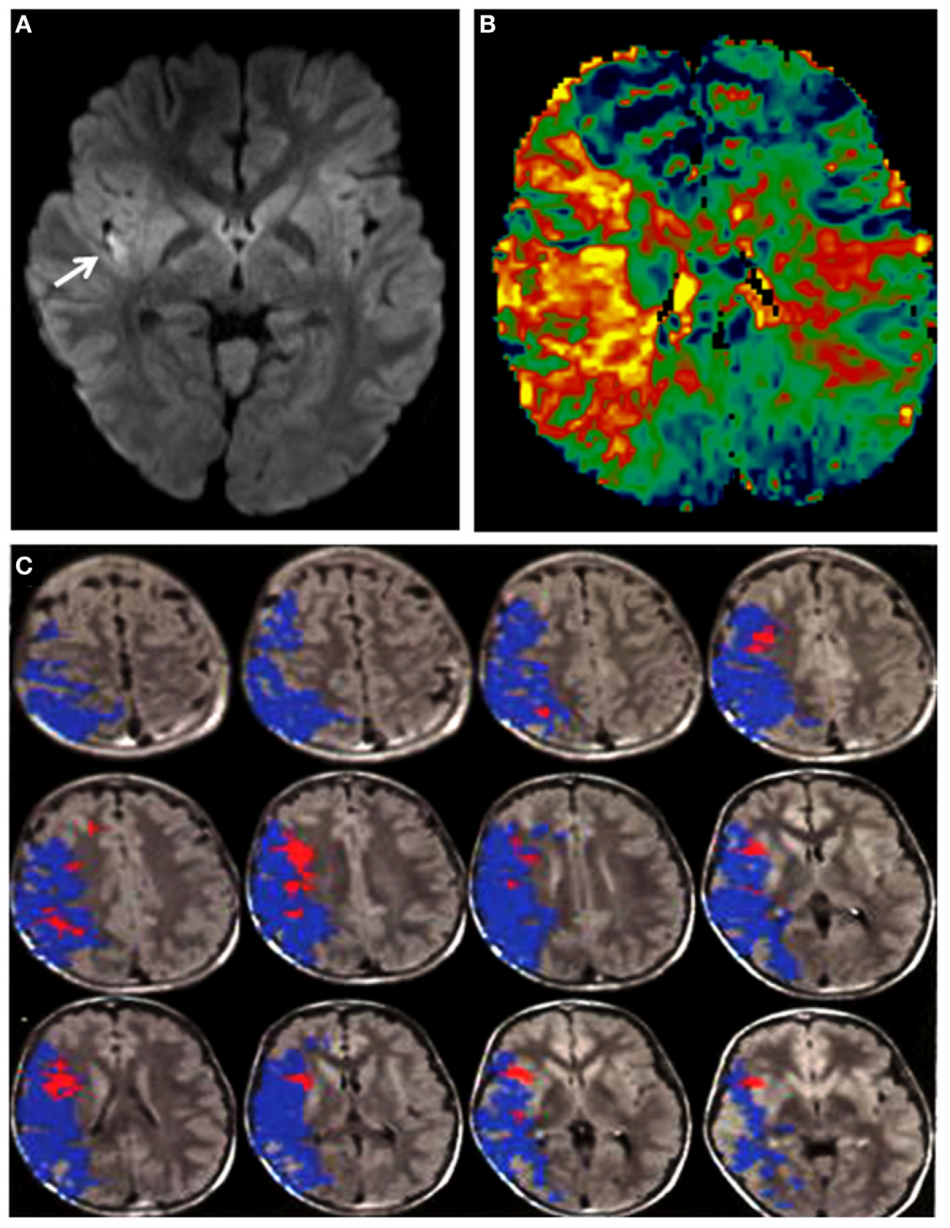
(A) DWI sequence showing a hyperintensity (arrow: infarct) in the right insula. (B) Perfusion imaging demonstrating increased mean transit time in the right middle cerebral artery (MCA) territory. (C) Magnetic resonance imaging with diffusion and perfusion-weighted sequences processed using Olea Sphere (Olea Medical Solutions, Inc., Cambridge, MA, USA) demonstrates volume of core infarct in red and hypoperfusion in blue, consistent with a large area of ischemic penumbra in the right MCA distribution.
